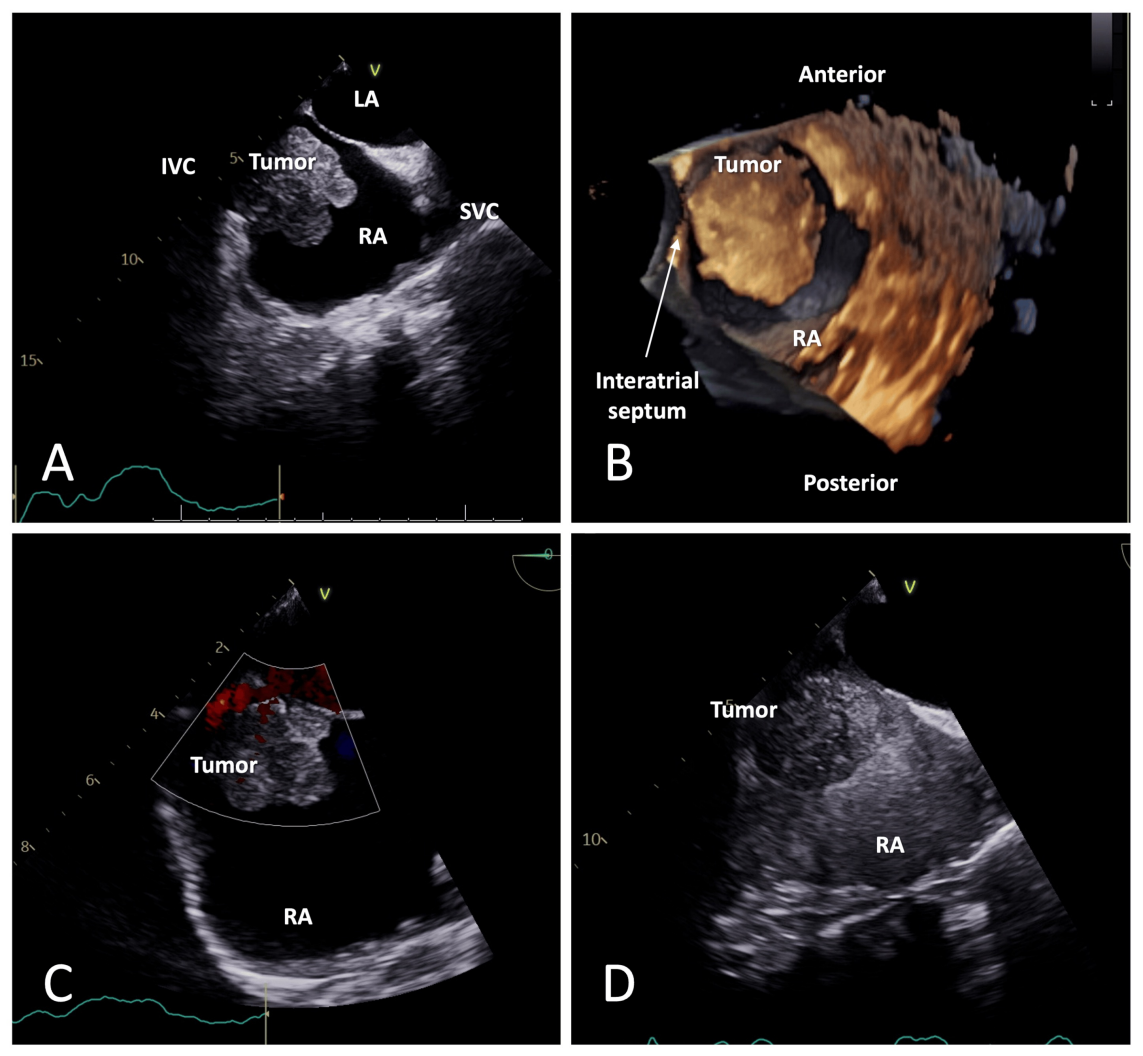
TEE evaluation of the RA mass (A) TEE evaluation demonstrating an RA mass projecting through the IVC in mid-esophageal bicaval view. (B) 3D imaging with post-processing demonstrating the RA mass in the RA *en face*. (C) Color flow interrogation without prominent vascularity. (D) Non-enhancing mass on contrast-enhanced echocardiography suggests a poorly vascularized mass. TEE: transesophageal echocardiogram; SVC: superior vena cava; IVC: inferior vena cava; RA: right atrium; LA: left atrium
